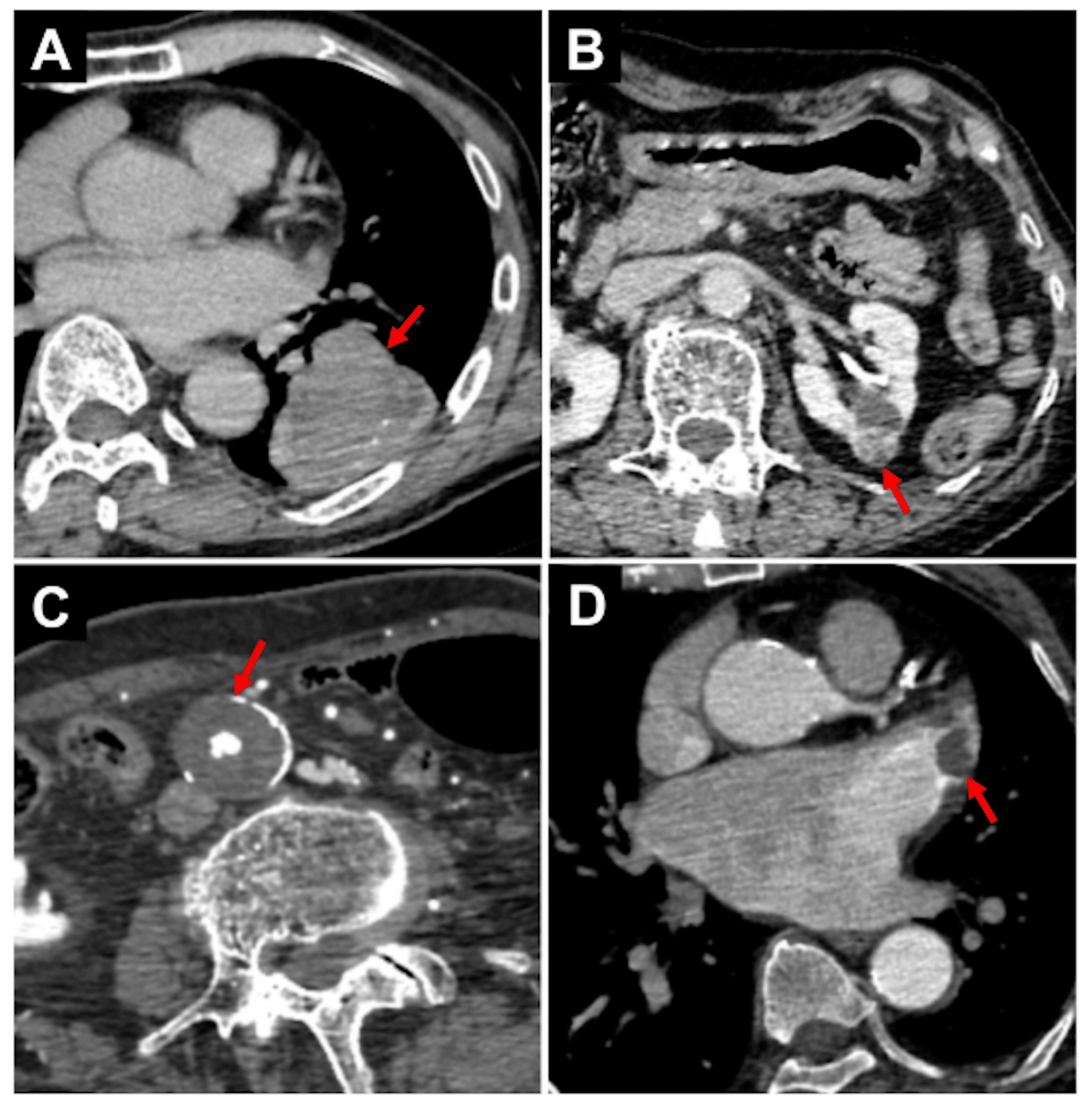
Representative cases of incidental findings on whole-body CTA Arrows indicate the abnormalities in each image. A) A suspected lung cancer lesion in the left lung of a 79-year-old man. B) A suspected kidney cancer lesion in the left kidney of a 91-year-old woman. C) A partially thrombosed abdominal aortic aneurysm in an 83-year-old man. D) A thrombus in the left atrial appendage of an 82-year-old woman. CTA, computed tomography angiography
